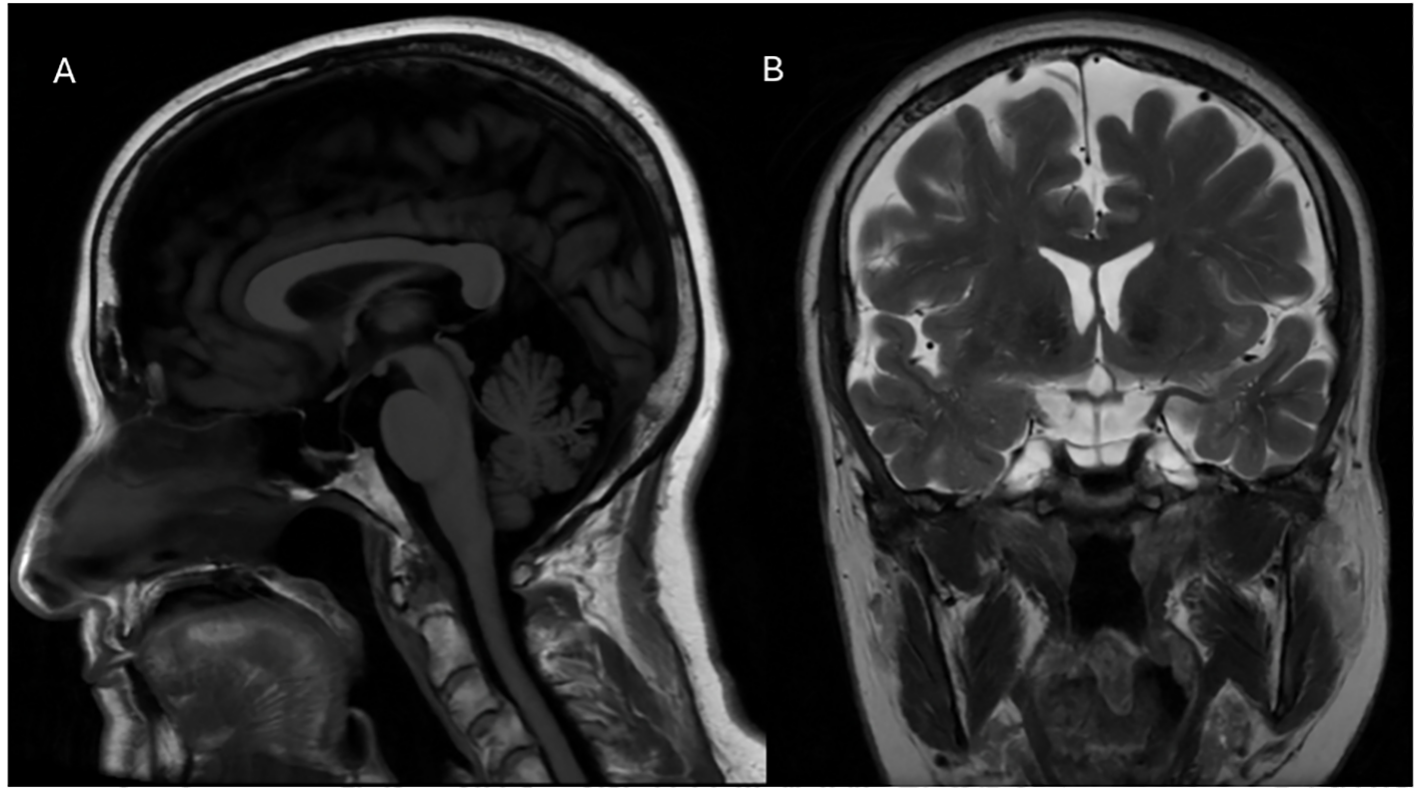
(A) Sagittal T1-weighted brain magnetic resonance imaging (MRI) sequence showing the findings of an partially empty sella turcica. (B) Coronal T2-weighted MRI sequence confirming the previously described findings.
